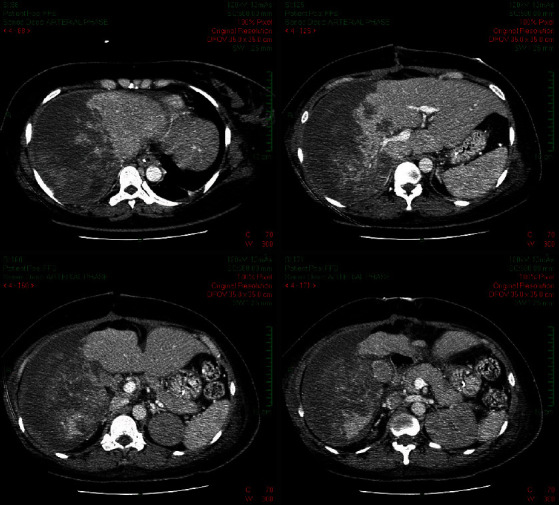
Perihepatic hematoma without signs of active bleeding on follow-up computed tomography.
